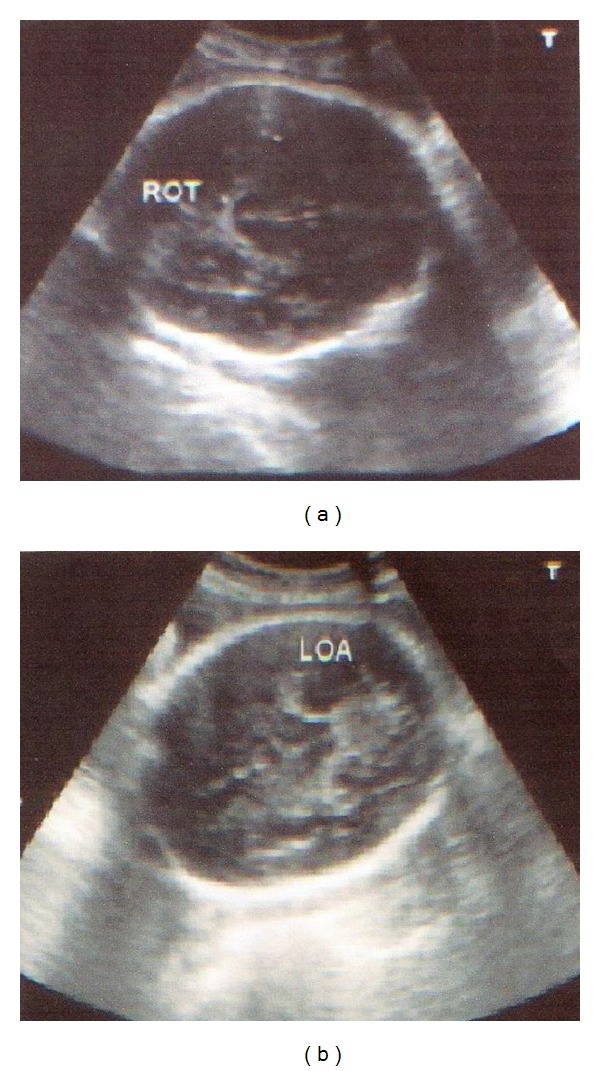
Transverse suprapubic transabdominal real-time ultrasound fetal head position assessment: (a) right occipitotransverse and (b) left occipitoanterior position.
